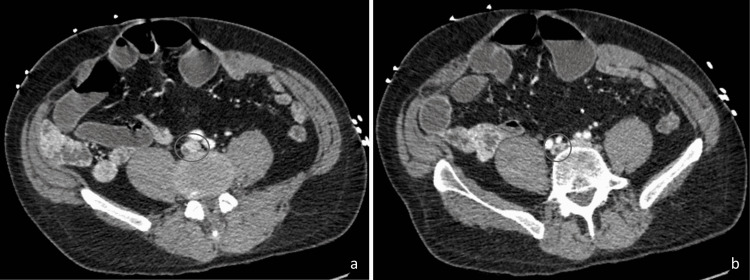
CT scan of the abdomen and pelvis with and without intravenous contrast demonstrating non-occlusive thrombi (circle) within the IVC (a) and the right common iliac vein (b) CT: computed tomography; IVC: inferior vena cava
